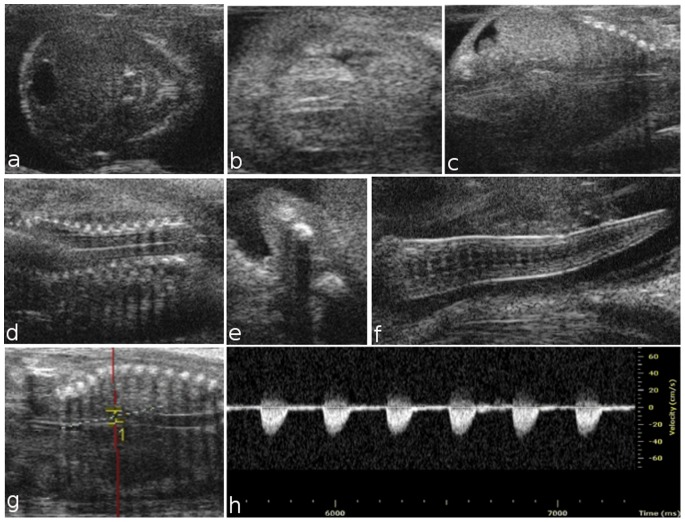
Ultrasound images of fetal development from embryonic day 14.5 to 16.5. At E14.5, progressive ossification is observed in the skull (a) and ribs (c), and the interventricular septum is completed (b). At 15.5, the development of the vertebral elements and humerus is complete (d, e). At E16.5, the curl is clearly visible (f). Dorsal aorta and corresponding Doppler spectral trace at E16.5, which exhibits a rapid upstroke and return to zero velocity during diastole (g, h).
